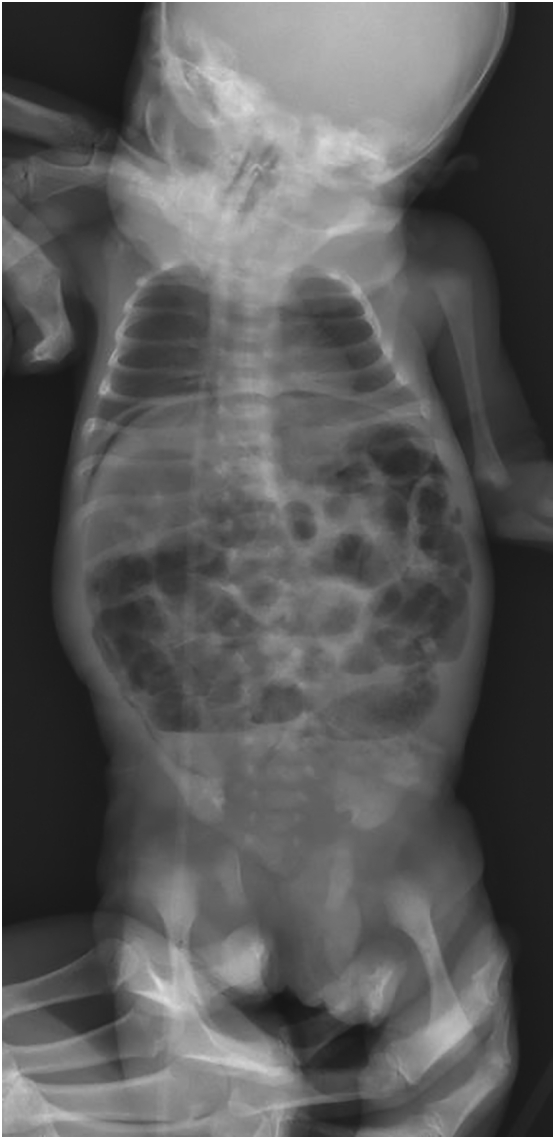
Free intraabdominal air on direct abdominal X-ray.
